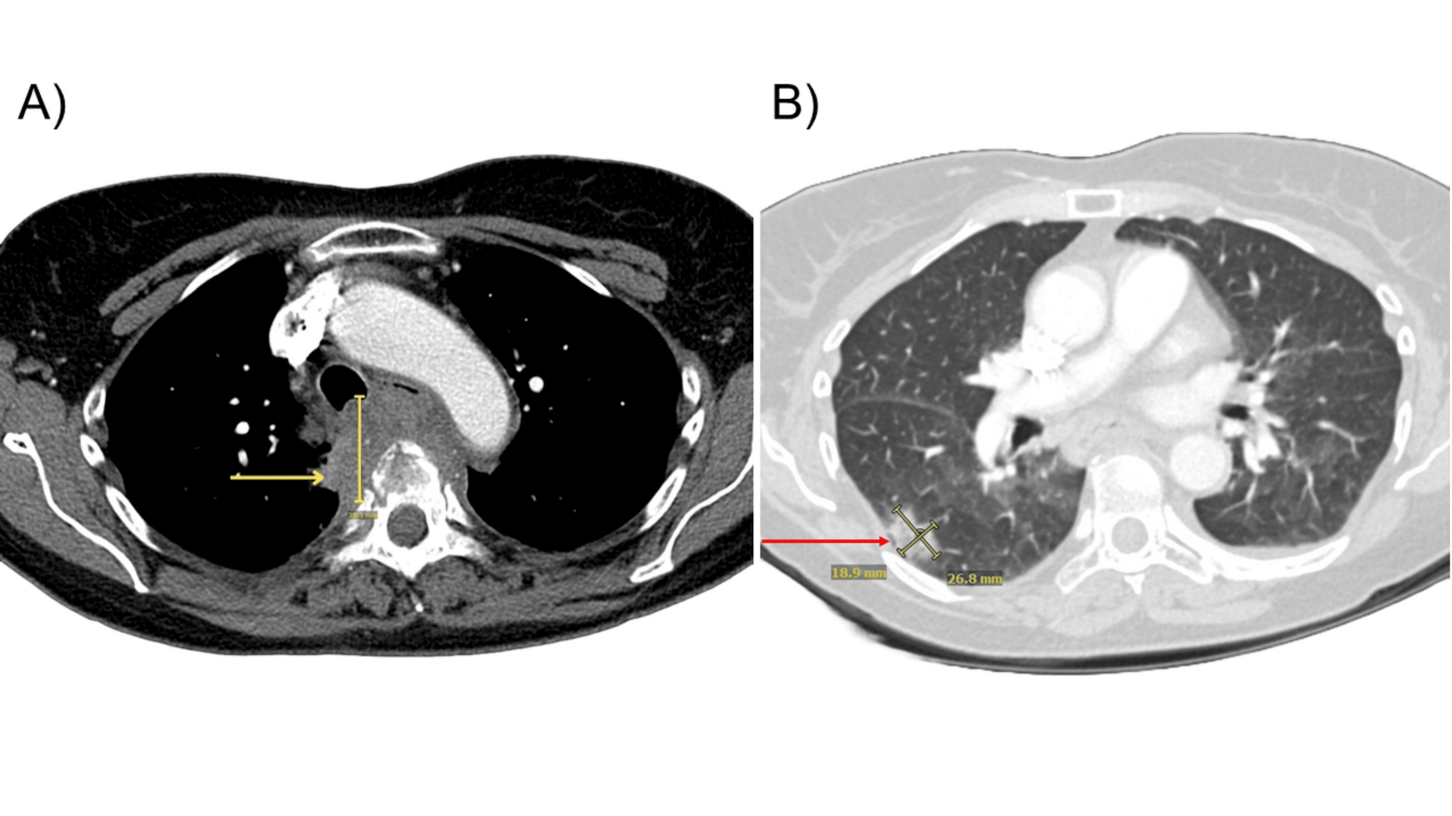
CT chest angiogram with contrast (A) Axial view from a contrast CT chest angiogram demonstrating an infiltrative soft tissue mass within the posterior mediastinum, with evidence of involvement of the adjacent vertebral bodies, suggestive of possible osseous invasion (noted by the yellow arrow). (B) Axial view of ground-glass nodular opacity noted in the right lower lobe, corresponding to an area of prior consolidation (noted by the red arrow). These findings raised concern for a possible underlying malignancy, particularly in the context of the patient's recent onset of thoracic back pain and persistent cough.
